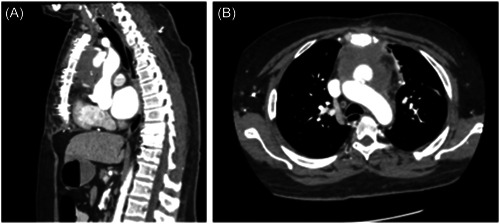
(A) Sagittal section demonstrating the enlarging aortic pseudoaneurysm. Of note is the relationship of the pseudoaneurysm neck in relation to the right main pulmonary artery on this image. This relationship was used as an anatomical landmark which was constant between CT and TEE imaging. This landmark was used to position the endoballoon intraoperatively as we knew the patent coronary grafts were more proximal (B) Axial CT images showing the proximity of the pseudoaneurysm to the posterior sternal table highlighting the risk of rupture upon re‐entry. CT, computed tomography; TEE, transthoracic echocardiogram.
